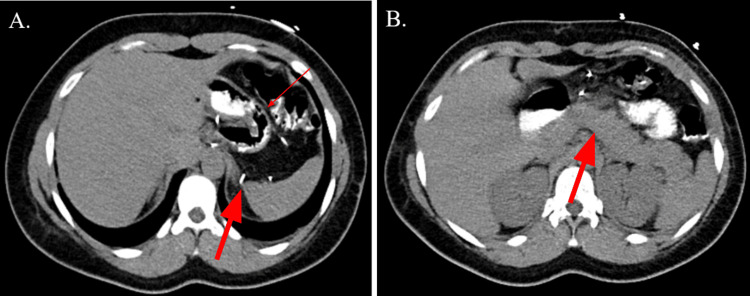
CT abdomen and pelvis with oral contrast (axial view) (A) Axial view of the abdomen demonstrating several surgical clips (thick arrow) and post-surgical changes (thin arrow) associated with sleeve gastrectomy. (B) Axial view of the abdomen at the level of the pancreas (thick arrow). No bowel obstruction or signs of acute inflammation were seen.
